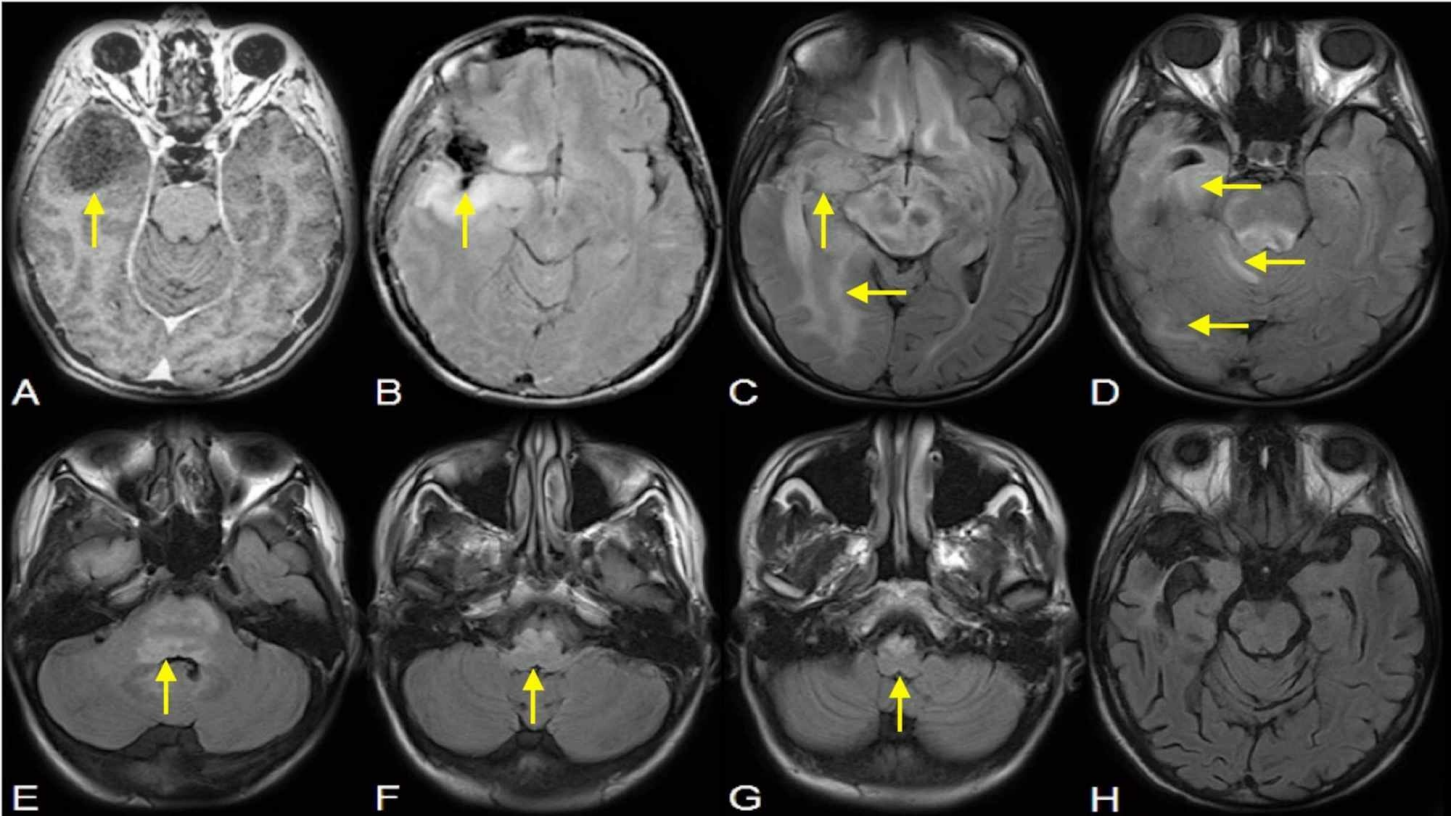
Gliomatosis Cerebri Radiographic Representation (A) Preoperative T1 postgadolinium magnetic resonance (MR) image showing right anterior temporal lobe mass. (B) Post-operative fluid-attenuated inversion recovery (FLAIR) image showing a residual tumor, in retrospect. (C-G) At 45 months follow-up, the lesion is now an obvious case of gliomatosis cerebri with tumor spread following multi-lobar mesolimbic system functional connections as well as both bilateral supratentorial and brain stem involvement. (H) Significant post-treatment diminution of multicompartmental mesolimbic gliomatosis with near-complete resolution of FLAIR hyperintensity in deeper brainstem structures thought to be compromised in central neurogenic hyperventilation (CNH).
